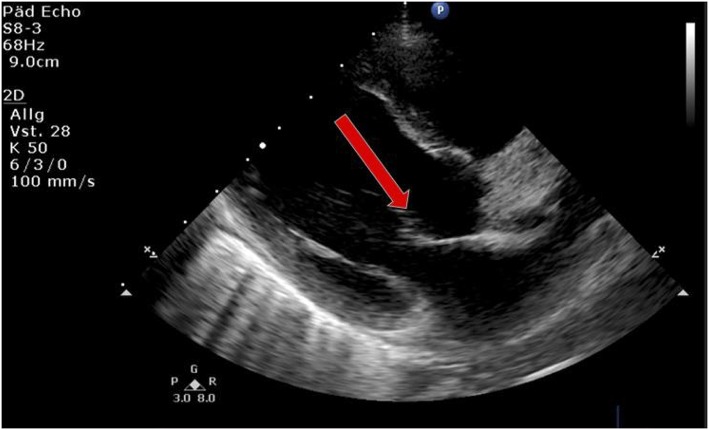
Echocardiography showing prolapse of mitral valve (arrow).
